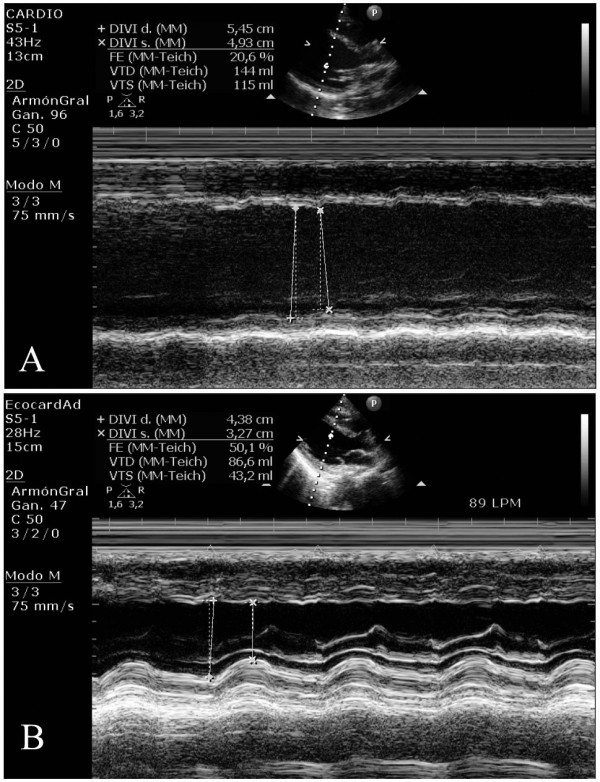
Ecocardiographic images: (A)  Transthoracic echocardiogram showing severe left ventricular dysfunction. (B) Normal LVEF after total recovery.
